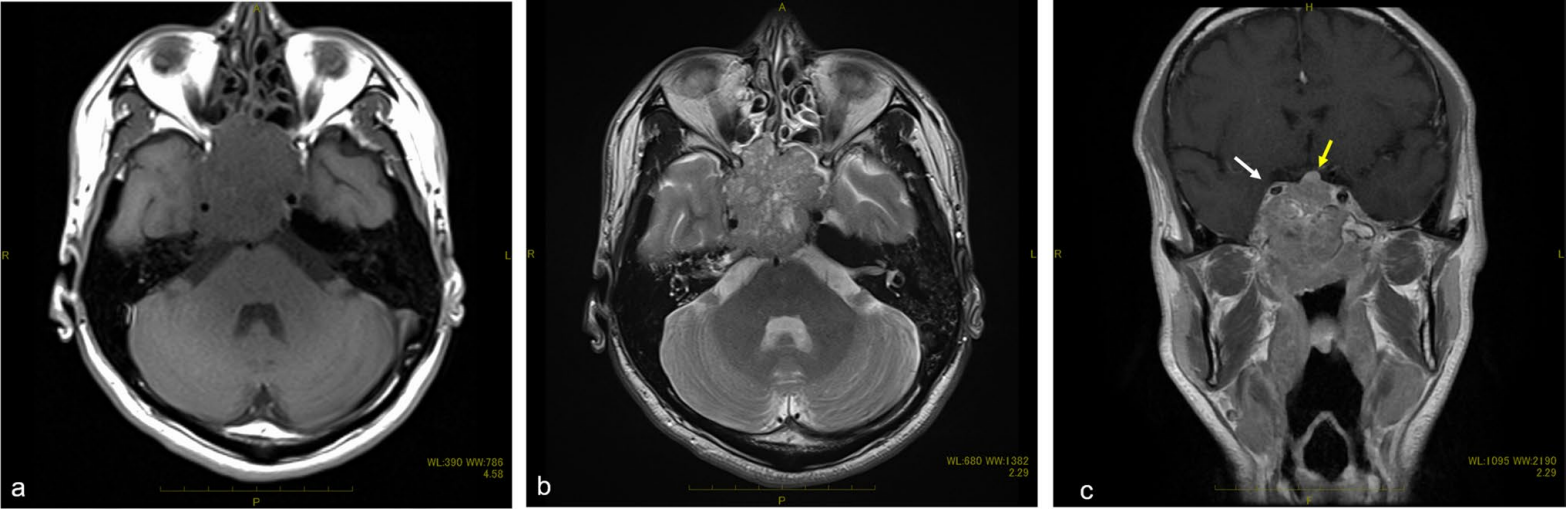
Magnetic resonance imaging: the tumor showed lower and intermediate intensity than the brain parenchyma on a T1W1 (a) and intermediate intensity compared to the brain parenchyma and with many high-intensity on a small cysts at T2WI (b). On the gadolinium-enhanced T1WI shoed a non-uniform enhancing tumor that compressed the pituitary gland (yellow arrow) and extended to the right spongy pulsation (white arrow) (c)
